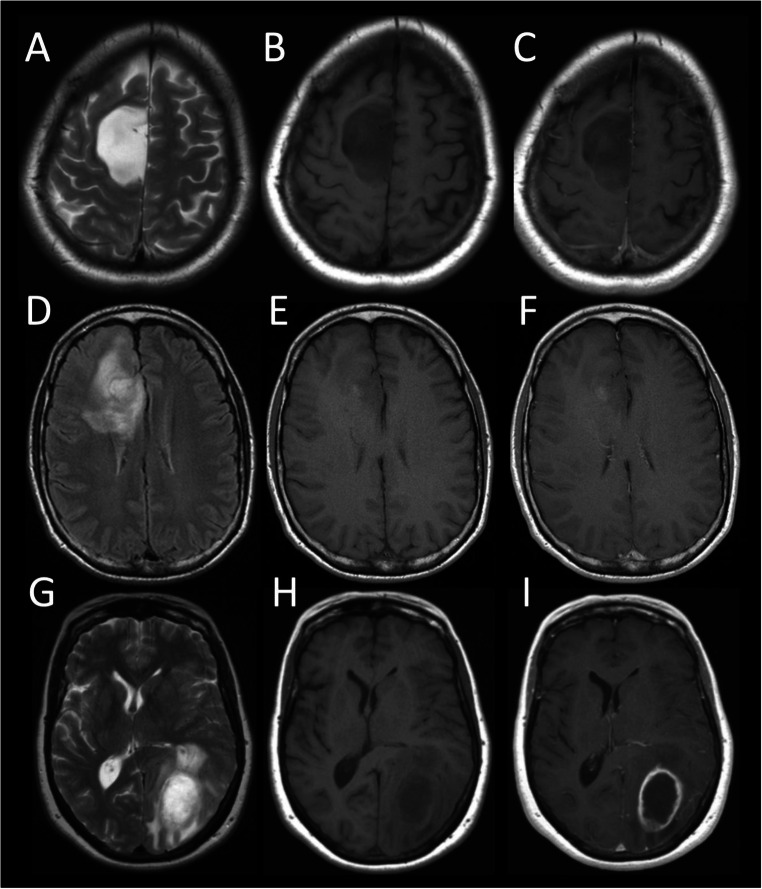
MR imaging in three different examples of adult-type diffuse gliomas
